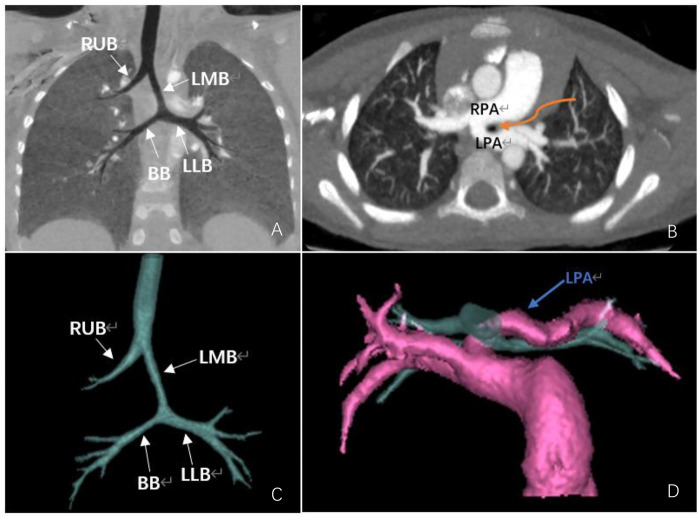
A 2-year-old male; preoperative cardiac CTA suggested pulmonary artery sling type 2A. (A) MinIP shows tracheal morphology and confirms the presence of a bronchial bridge. (B) Axial MIP showed an abnormal origin of the left pulmonary artery from the right pulmonary artery, with corresponding tracheal compression and narrowing (curved arrow). (C,D) Volume rendering and reconstruction showed tracheal morphology and the relationship between the trachea and pulmonary artery. RUB, right upper lobe bronchus; BB, bridging bronchus; LLB, left lower lobe bronchus; LMB, left main bronchus; LPA, left pulmonary artery; RPA, right pulmonary artery.
